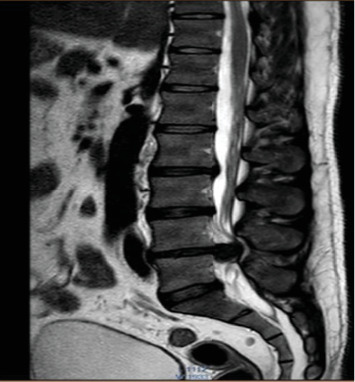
T2-weighted MRI showing posterior disc bulge at L4-L5 level with extrusion leading to severe narrowing of the spinal canal with compression of cauda equina nerve root.
